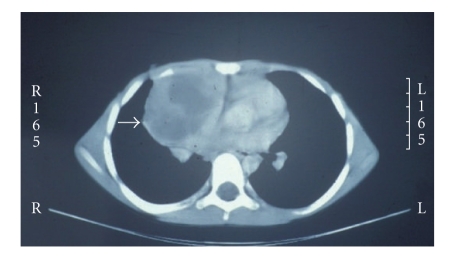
CT scan demonstrating a large cystic anterior mediastinal mass (arrow).
